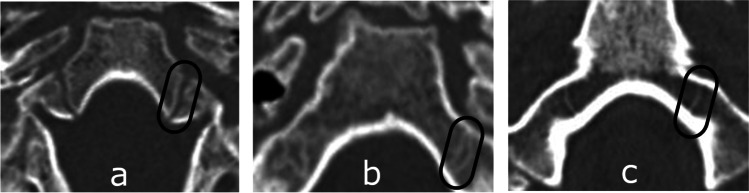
Computed tomography images depicting consecutive stages of anterior intra-occipital suture ossification: A Stage 1 – no ossification; B Stage 2 – partial ossification; C Stage 3 – complete ossification
